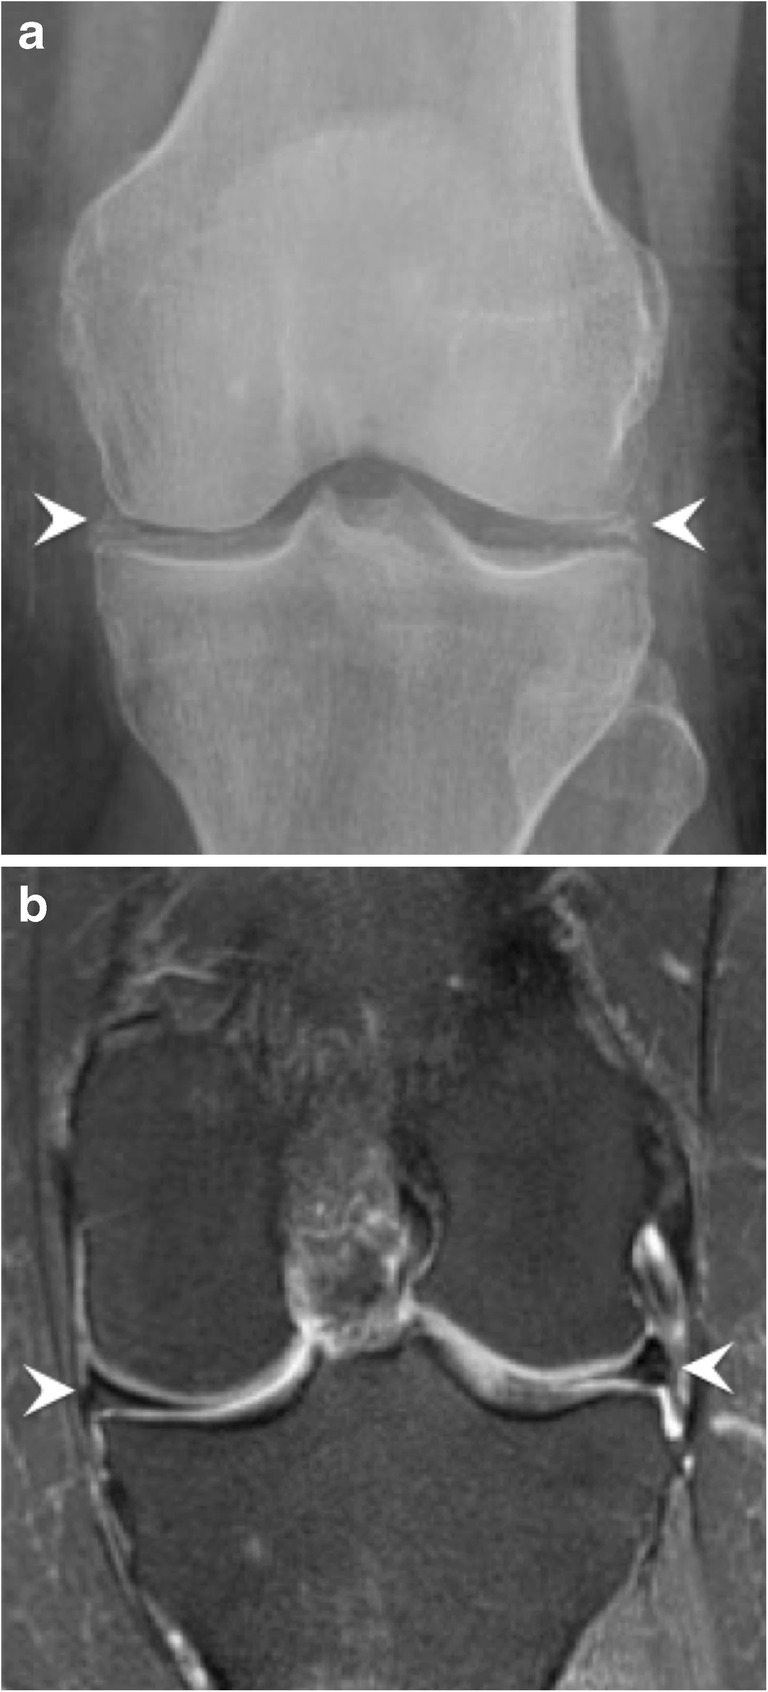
Chondrocalcinosis in the knee. (a) Anteroposterior radiograph of the left knee and (b) coronal fat-saturated proton density (PD)-weighted MRI image show chondrocalcinosis in the menisci (arrowheads)
